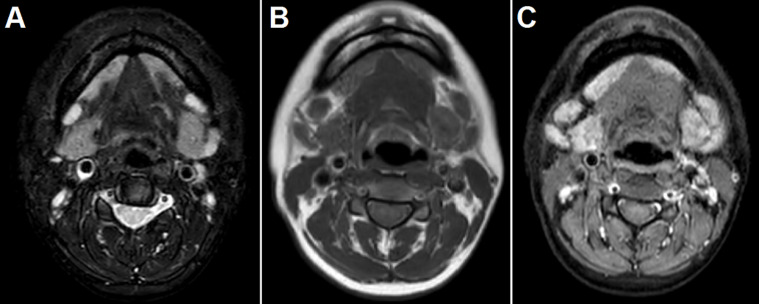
facial MRI before treatment: A) fat-suppressed T2-weighted images showed that the submandibular glands were diffusely enlarged and hypointense; B) T1-weighted images demonstrated the low signal intensity of submandibular glands; C) T1-weighted images with contrast showed the remarkably homogeneous enhancement of these glands
